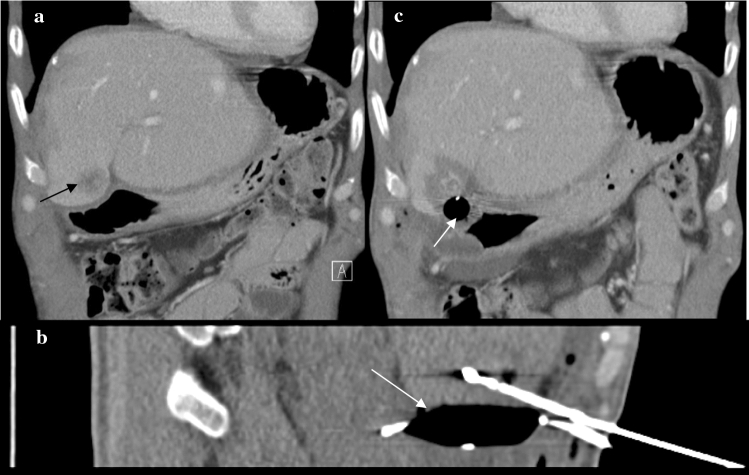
a Preinterventional CT-scan of a 56-year old male patient previously treated by right hemihepatectomy with a new liver metastasis (black arrow) from rectal carcinoma in segment IVb adjacent to the stomach. b Positioning of the MW antenna following interposition of an angioplasty balloon (white arrow) between the liver and the stomach. c Postinterventional CT-scan showing the balloon in between the ablation zone and the stomach wall
